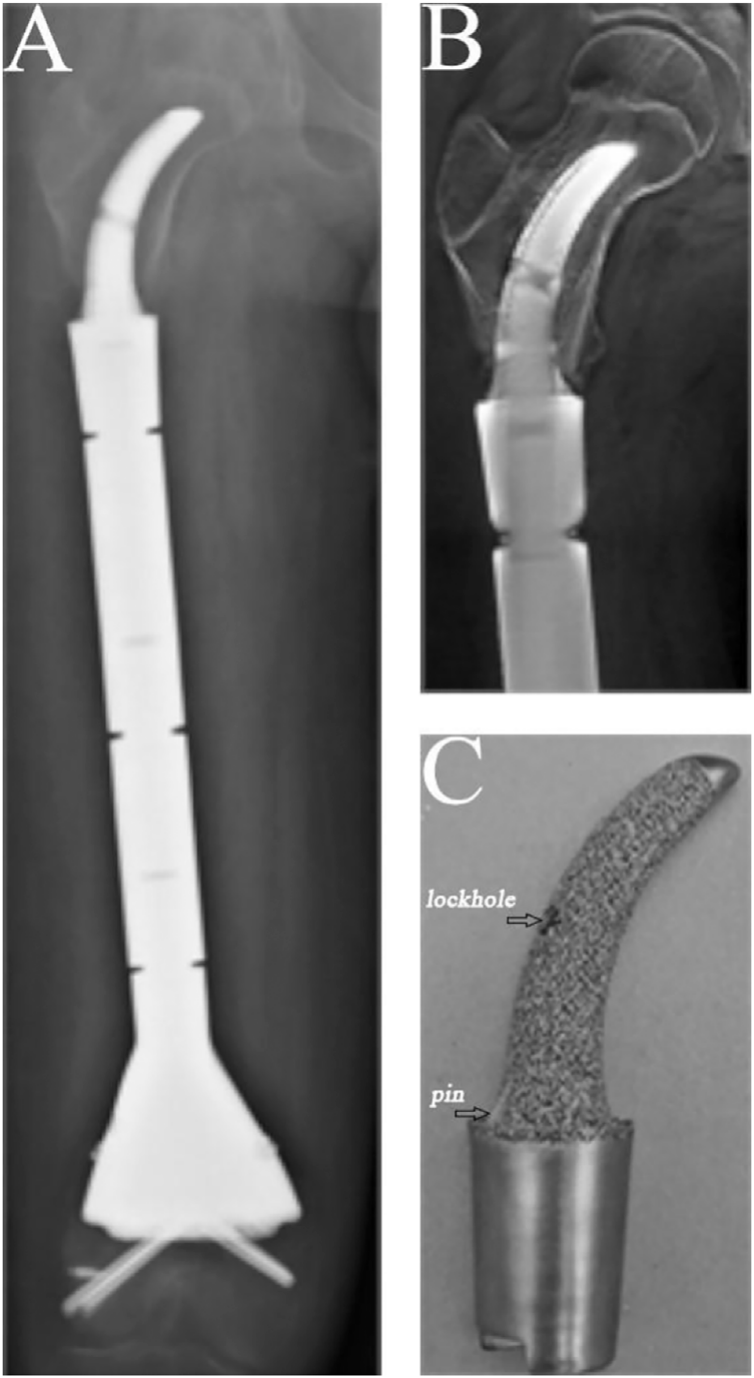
A case of reconstruction of the femoral diaphysis following femoral diaphyseal resection of 73% of the length of the femur. (A) Posteroanterior radiograph of the entire femur. (B) Posteroanterior T-smart view of the stem insertion region of the femur. (C) Gross appearance of the curved stem.
